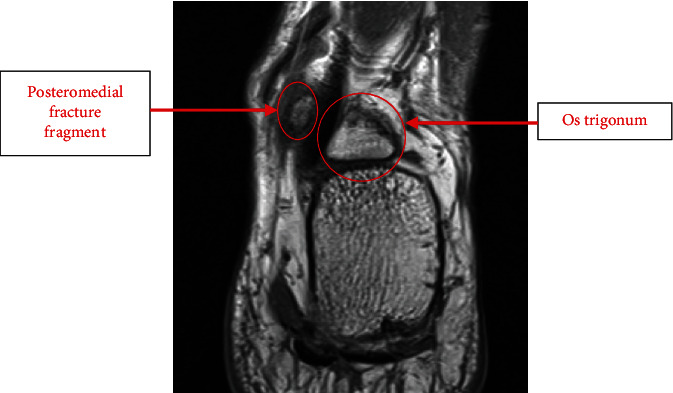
Coronal slice of T2 MRI demonstrating the large os trigonum lateral to the FHL tendon and the fracture fragment medial to the FHL tendon.
